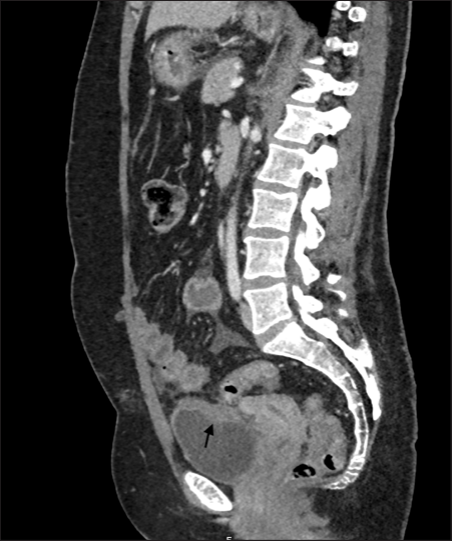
A sagittal reformatted CT image shows a high-attenuating clot abutting on the bladder dome, the so-called « sentinel clot sign ».
